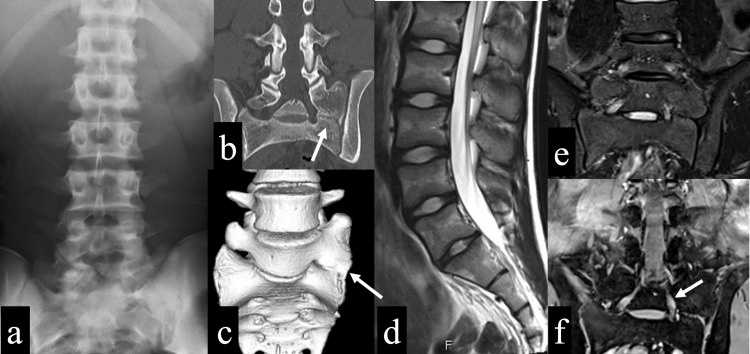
Imaging findings at the initial examination a: Plain X-ray anteroposterior view showed an enlargement of the left transverse process of the sixth lumbar vertebra. b: CT coronal view showed the left transverse process of the sixth lumbar vertebra articulating with the sacral alar and iliac bone (white arrow). c: CT 3D image showed that the left transverse process of the sixth lumbar vertebra was continuous with the sacral alar (white arrow). d: MRI T2-weighted sagittal view showed no intervertebral disc degeneration. e: MRI STIR coronal view showed no fatigue fracture of the sacral alar. f: MRI T2-weighted coronal view showed abnormal left S1 nerve root migration (white arrow).
